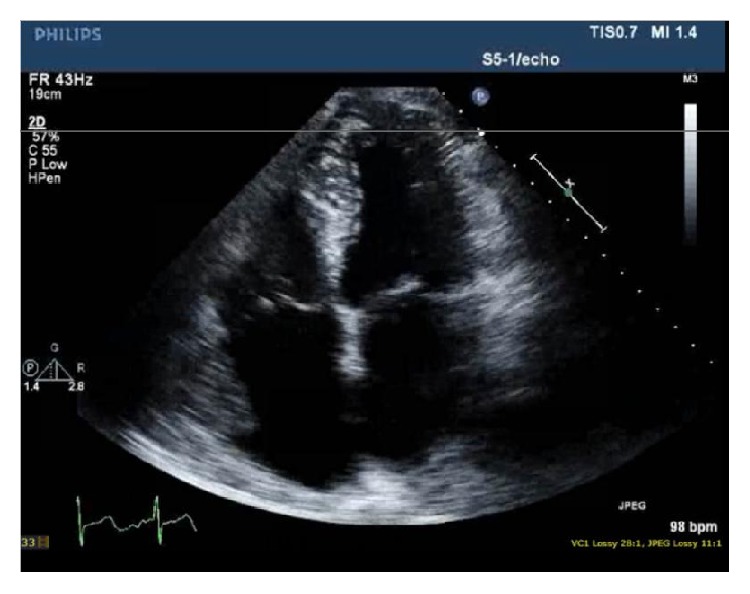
Apical 4 chamber view of 2D echocardiogram showing right atrial mass/clot.
